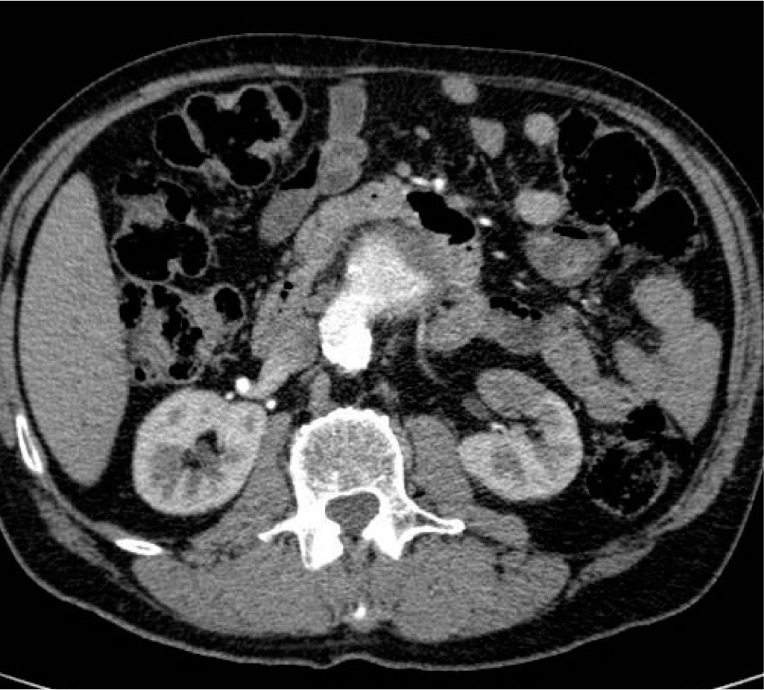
More proximal computed tomography demonstrating left accessory renal artery arising from origin of abdominal aortic aneurysm that was supplying the lower pole tumour, the superior aspect of which can also be visualised
